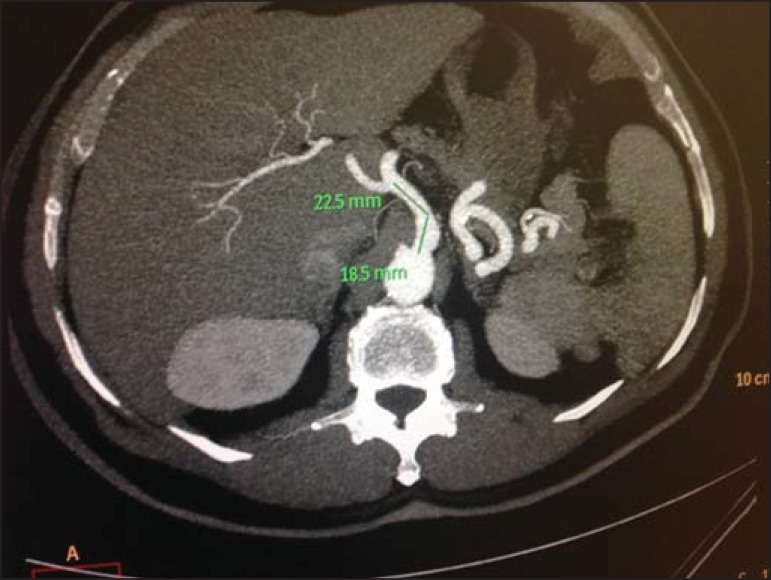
Axial, contrast-enhanced CT showing the longer length of the celiac trunk
